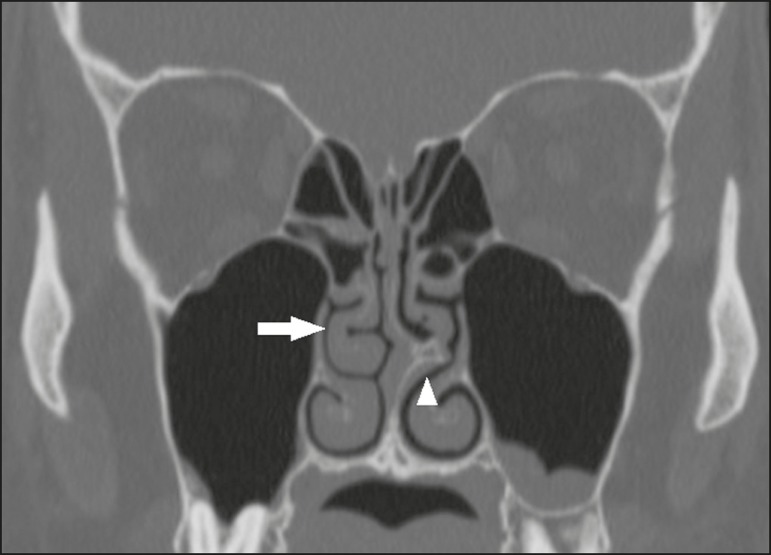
Coronal CT slice, with bone window settings, showing a paradoxical right
